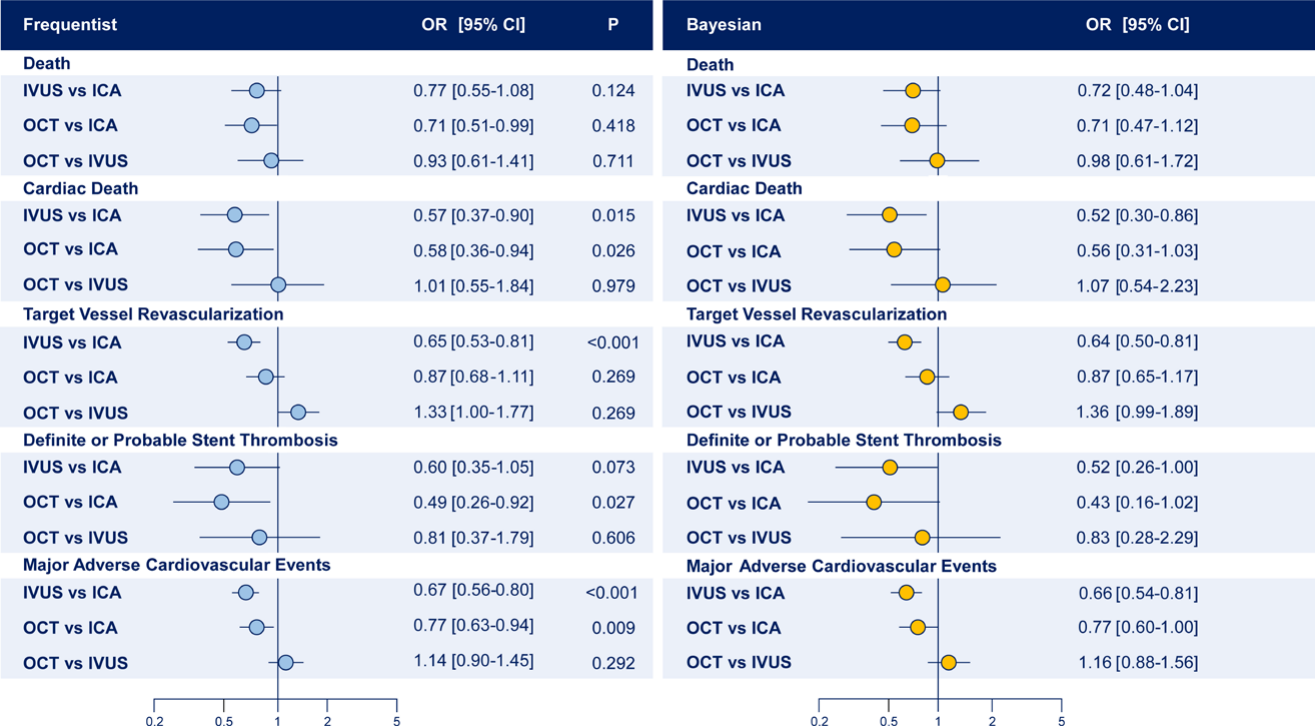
Secondary outcomes: network meta-analyses. Secondary outcomes across comparisons as assessed by frequentist (left, light blue) and Bayesian (right, yellow) network meta-analyses. CrI indicates credible interval; ICA, invasive coronary angiography; IVUS, intravascular ultrasound; OCT, optical coherence tomography; and OR, odds ratio.
